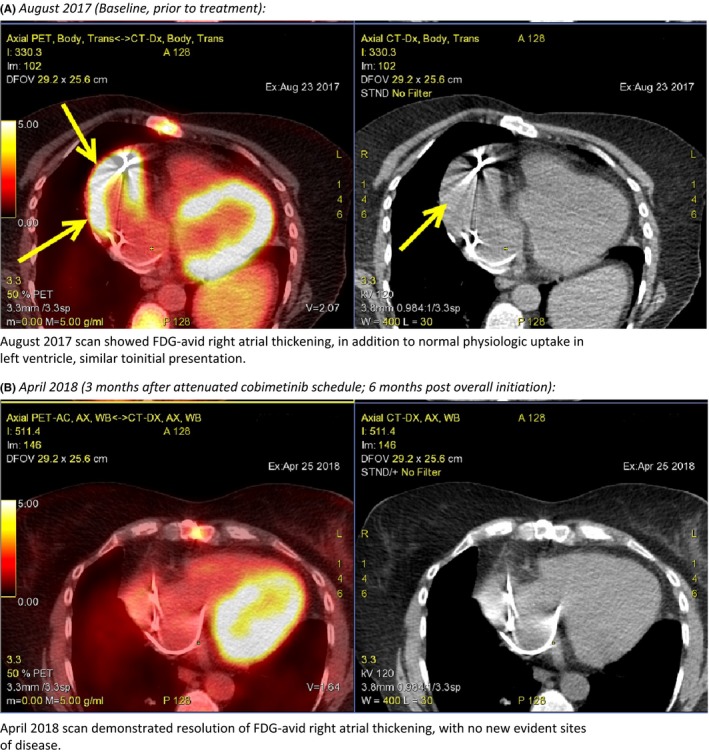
Radiographic demonstration of disease response (PET CT). A, August 2017 (Baseline, prior to treatment): August 2017 scan showed FDG‐avid right atrial thickening, in addition to normal physiologic uptake in left ventricle, similar to initial presentation. B, April 2018 (3 mo after attenuated cobimetinib schedule; 6 months post overall initiation): April 2018 scan demonstrated resolution of FDG‐avid right atrial thickening, with no new evident sites of disease
